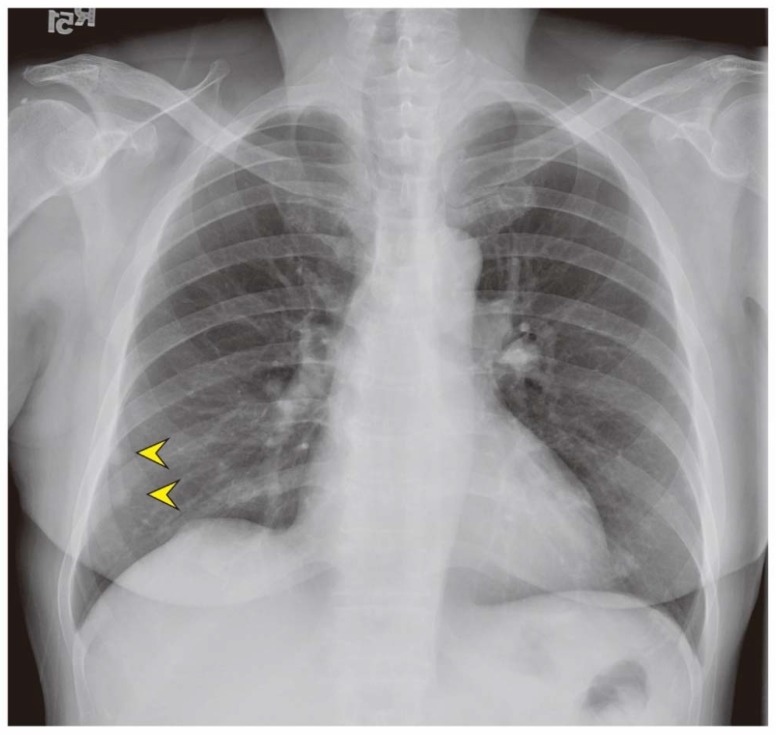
The chest X-ray revealed two homogeneous pulmonary nodules at right lower lung field (arrow head).
